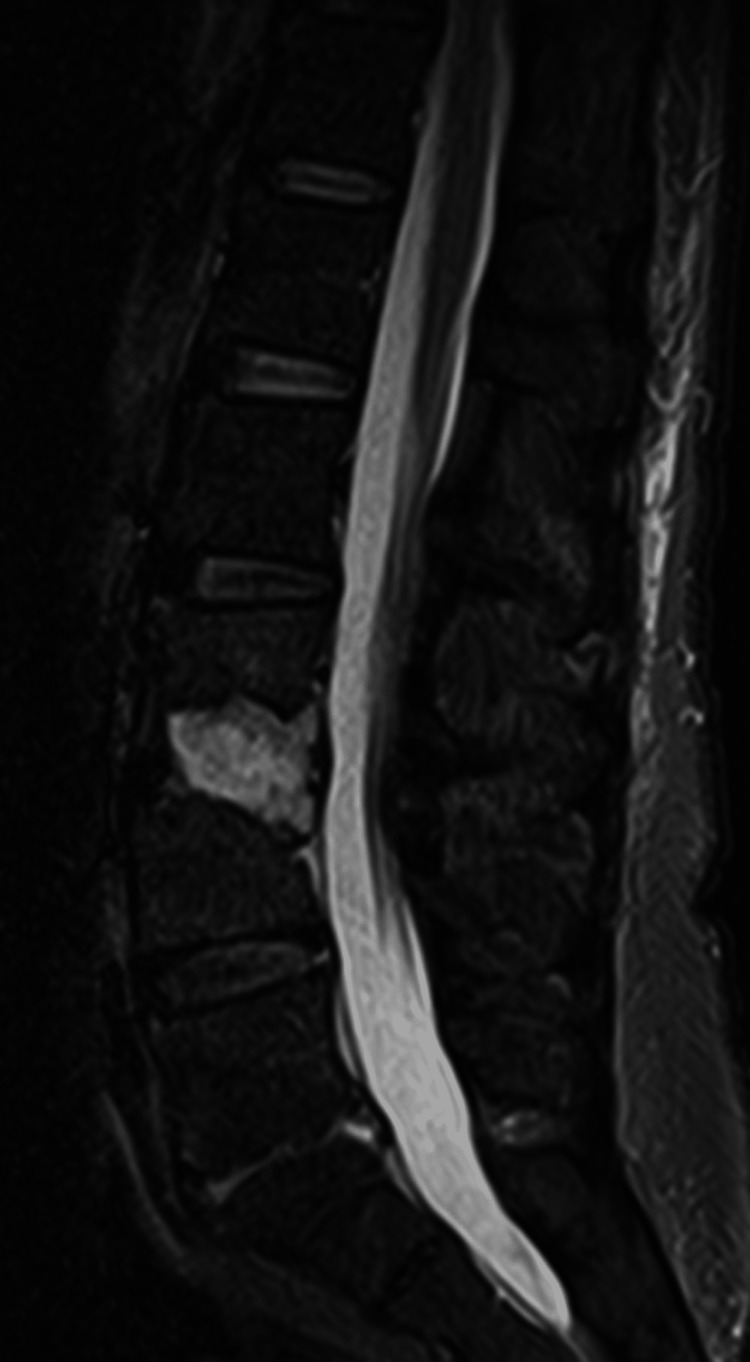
MRI six weeks after the start of the treatment (sagittal view).
